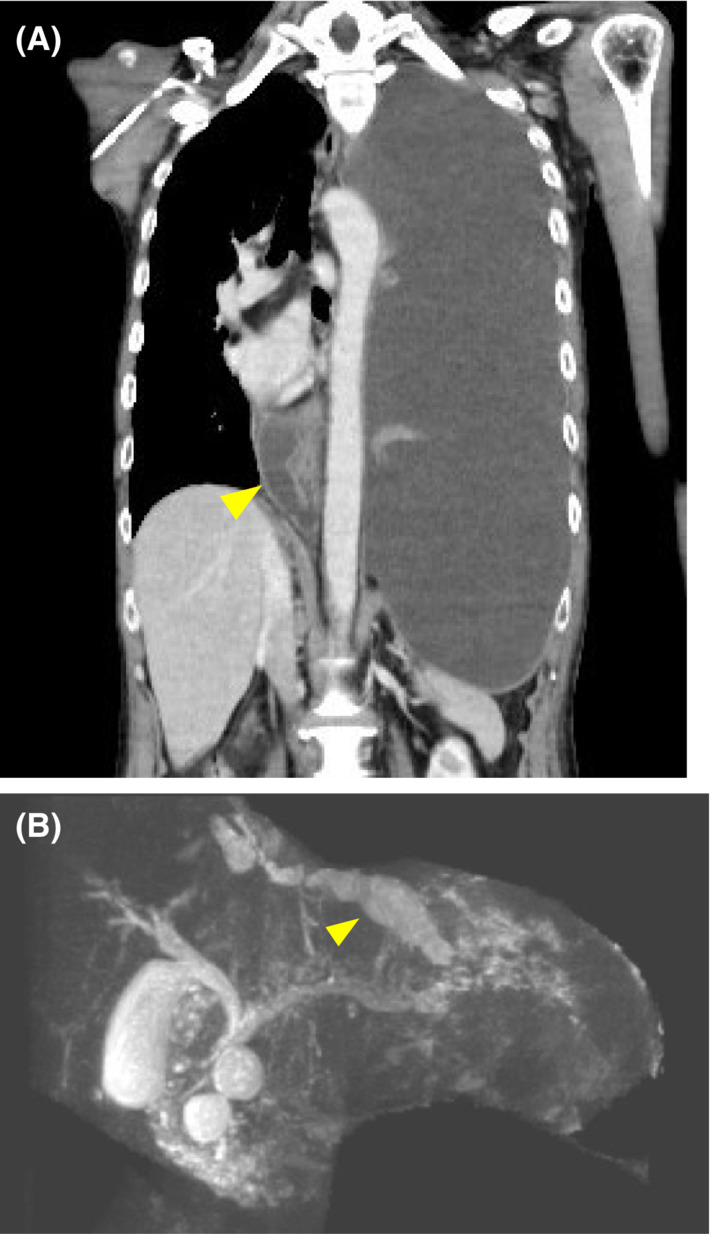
Contrast‐enhanced computed tomography (A) and plain magnetic resonance cholangiopancreatography (B) showed a pancreatic tail pseudocyst extending and connecting to the left pleural cavity through a mediastinal esophageal hiatal hernia (arrowheads).
